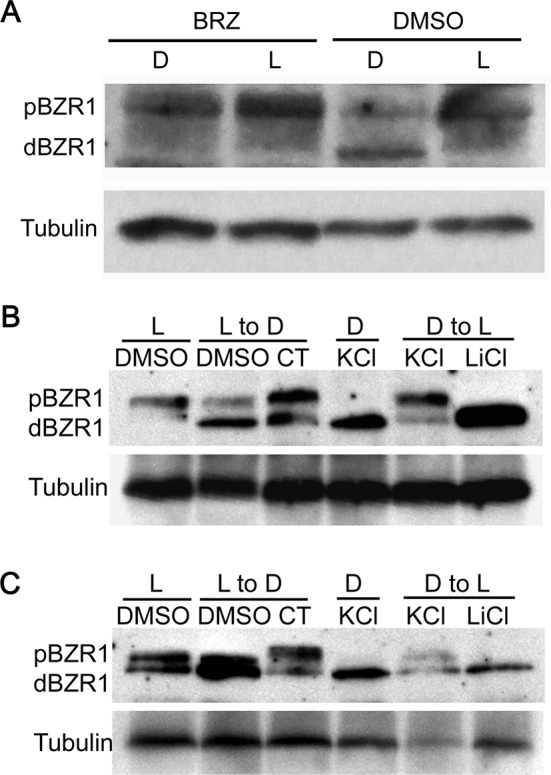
Light-regulated phosphorylation change of BZR1 requires the existence of endogenous BR as well as functional BIN2 and PP2A (A) Dark could not induce BZR1 dephosphorylation in the absence of endogenous BR. To eliminate the endogenous BR, seeds were germinated and grown in MS medium supplied with BRZ (2 µM) or its mock under a 16 h/8 h light/dark cycle for 6 days. Then, the 6-day-old seedlings were treated with light or dark for another 20 h. (B and C) CT treatment alleviated the dark-induced BZR1 dephosphorylation and LiCl treatment abolished the light-induced BZR1 phosphorylation in the *pBZR1::BZR1-CFP* seedlings (B) and *pBZR1::mBZR1-CFP* seedlings (C) respectively. Tubulin was used as loading control in (A), (B), and (C).
